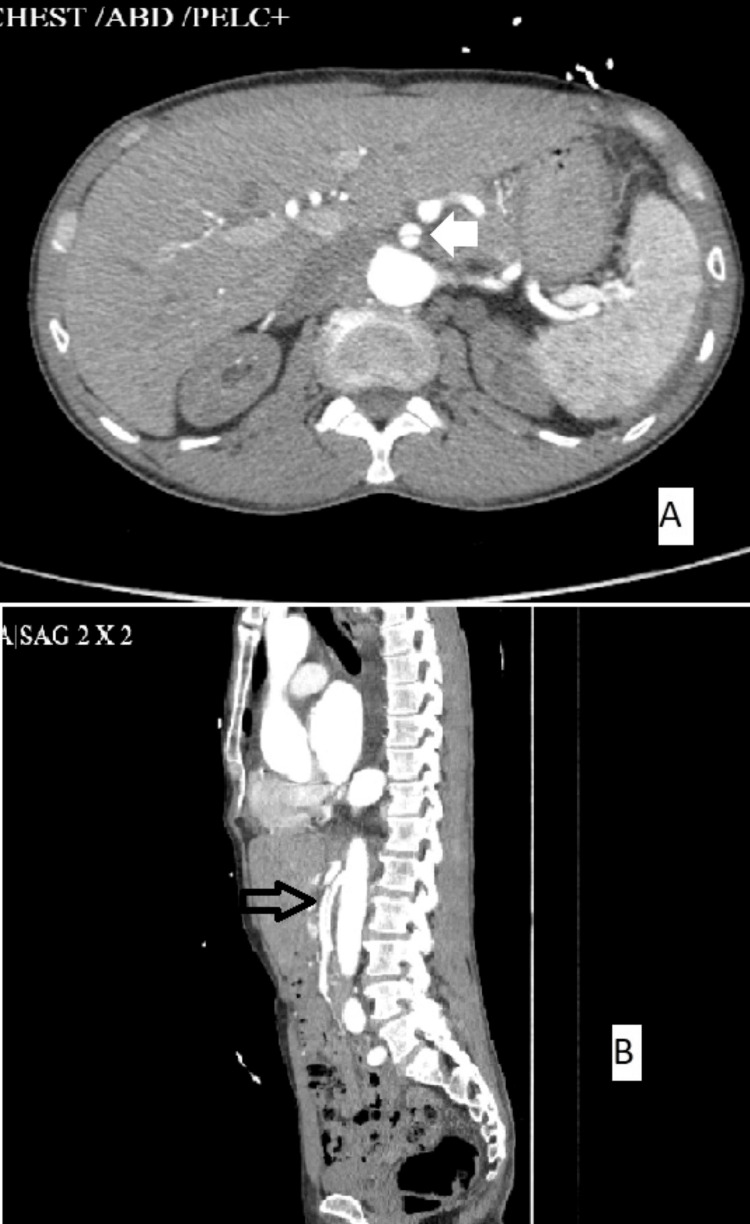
Computed tomography angiography of the abdomen showing dissection of the superior mesenteric artery (white arrow in image A and black arrow in image B).
